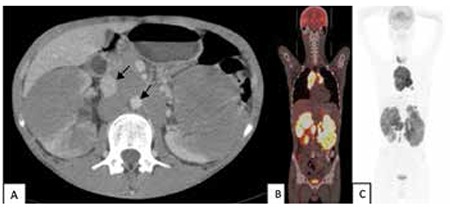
Computed tomography with intravenous contrast reveals enlargement of both kidneys with bilateral renal masses and paraaortic, paracaval lymph nodes (arrows) (A); 18F-fluorodeoxyglucose positron emission tomography-computed tomography fusion images showed very intense diffuse fluorodeoxyglucose uptake in bilaterally enlarged kidneys (B); maximum intensity projection images of positron emission tomography-computed tomography scan demonstrated multifocal increased 18F-fluorodeoxyglucose uptake in the thyroid, mediastinum, and kidneys (C).
